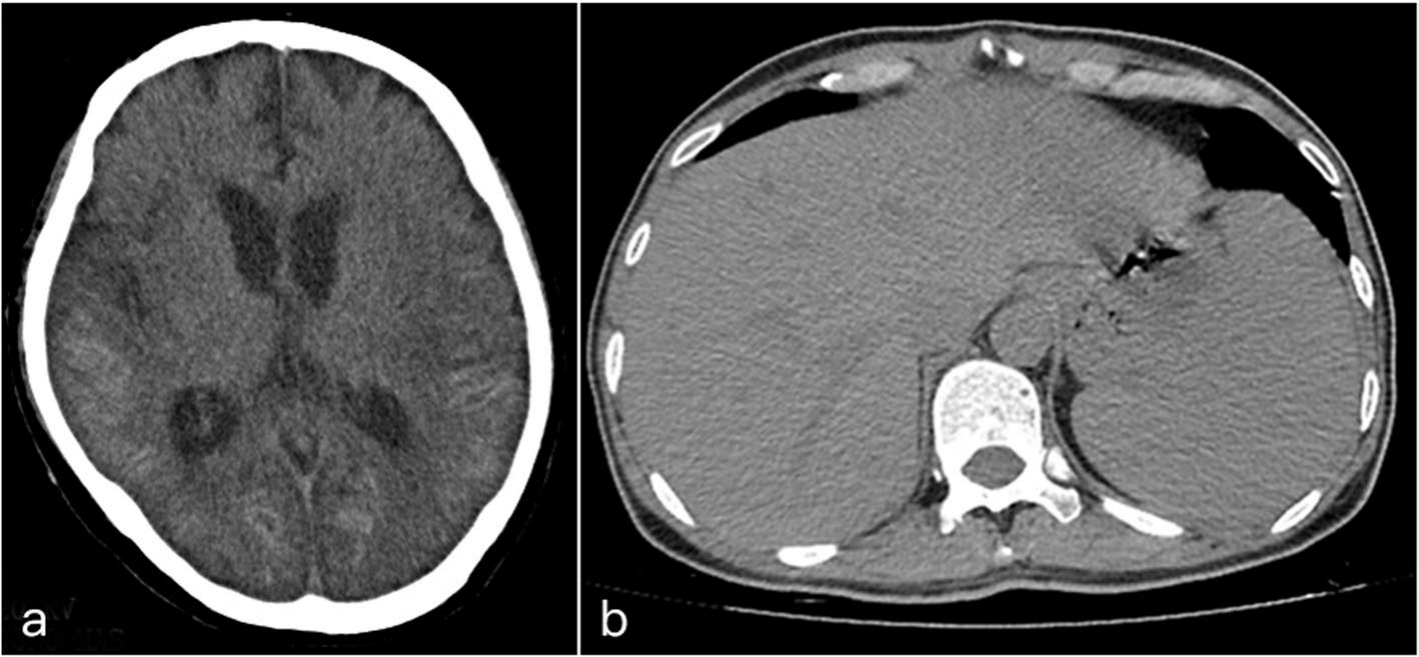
Manifestation of cranial CT imaging of the patient No.1 (a) [9], and manifestation of abdominal CT imaging of patient No.6 (b)
